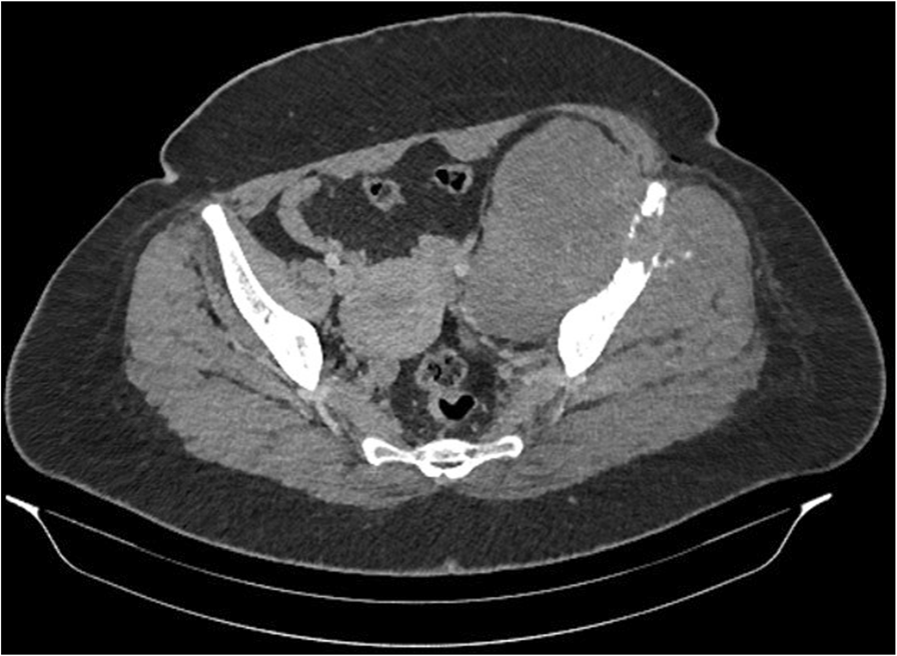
Axial computed tomography showing a solid aggressive osteolytic mass of the left iliac bone with extensive soft tissue invasion
